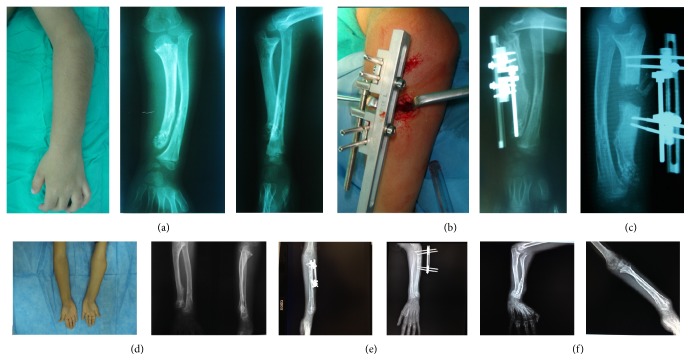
The preoperative, intraoperative, and postoperative images of the first case. (a) Preoperative and (b) intraoperative images of the first time of distraction, (c) 45 days of distraction osteogenesis, (d) clinical and radiographic images of patients after 2 years of distraction osteogenesis, (e) postoperative images of patients after second distraction osteogenesis, and (f) images of patients after implants were removed.
